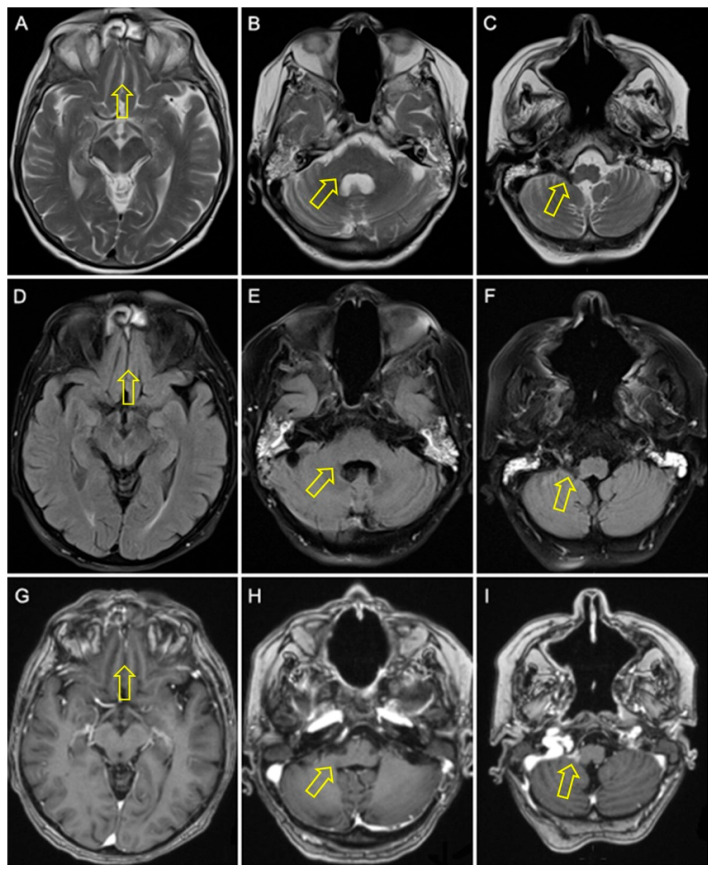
The patient received pulse methylprednisolone therapy (total 1.25 g) and a single pulse dose (0.5 g/m^2^) of cyclophosphamide (CYC). The treatment was continued with rituximab (RTX) at a dose of 375 mg/m^2^ administered in four weekly doses, leading to significant clinical improvement. A follow-up brain MRI performed in January 2025 revealed significant regression, with small residual lesions frontobasally bilaterally and in the right cerebellomedullary cistern. MRI revealed discrete irregular frontobasal meningeal thickening (A,D,G—yellow arrow), and small irregular lesion in the region of the right middle cerebellar peduncle (B,C,E,F,H,I—yellow arrow), without T2W (A–C) and FLAIR (D–F) hyperintensity, but with discrete postcontrast enhancement (G–I). Standard induction with corticosteroids and CYC is effective in most GPA patients with CNS involvement [5]. However, in refractory or relapsing cases, RTX has shown superior efficacy [5]. Therapy was continued with low doses of prednisone and methotrexate (0.3 mg/kg BW weekly), with no new neurological symptomatology observed during the follow-up period. In July 2025, the patient experienced worsening of respiratory symptoms, including a persistent cough and new-onset hemoptysis. Lung CT showed active lung involvement with formation of caseous granulomas (Figure 2, Images 4a–4f). The patient received two pulse doses of intravenous methylprednisolone (500 mg each), followed by RTX at a dose of 1000 mg administered twice, two weeks apart. The treatment resulted in a good clinical response, with sustained disease remission to date. This case highlights the diagnostic challenge posed by intracerebral granulomas as a rare manifestation of GPA in a patient with unremarkable ANCA serology. The tumor-like appearance of intracranial lesions on MRI may lead to significant diagnostic delay. Multimodal imaging, integrating brain MRI with sinonasal and pulmonary CT findings, proved essential in establishing the correct diagnosis. This case reinforces the importance of including GPA in the differential diagnosis of intracranial mass lesions and underscores that early initiation of immunosuppressive therapy is critical to prevent life-threatening compressive complications.
